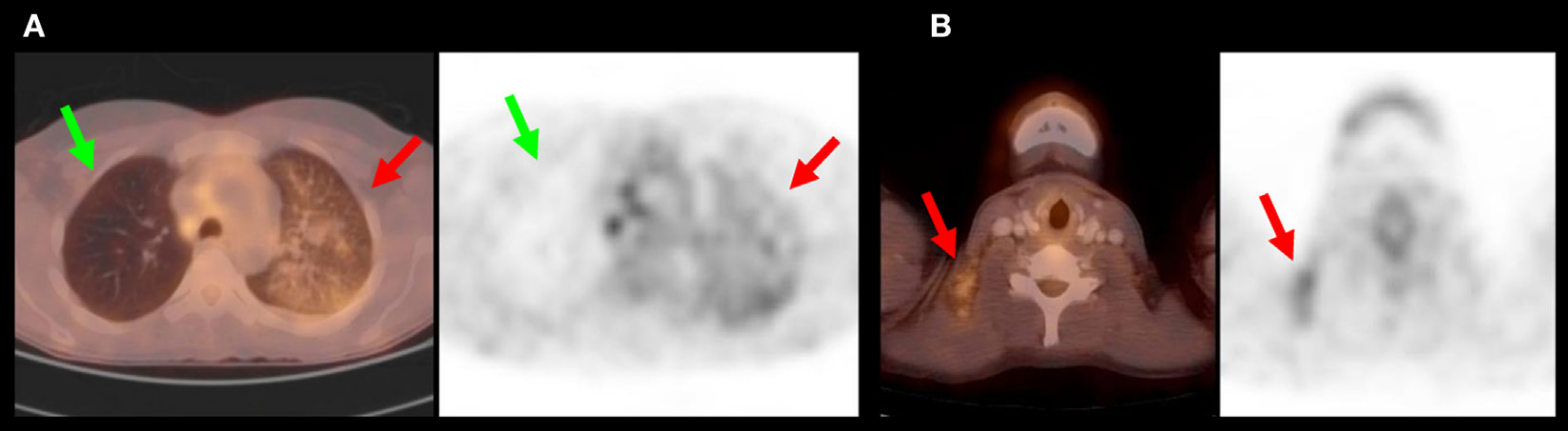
This 36-year-old woman had a thrombosis of her left leg due to a coagulopathy and suffered multiple embolic ischemic strokes. Neither ultrasound of the carotid arteries, cardiac ultrasound, nor cerebral angiography revealed any causative pathology. (A) PET/CT revealed a diffuse, blotchy enhancement of the left lung suggestive of a bronchoalveolar carcinoma (red arrows). Green arrows depict the healthy right lung. The PET/CT overlay is shown on the left side, with the conventional PET-scan on the right. (B) Multiple suspicious lymph nodes were identified in the neck. A biopsy revealed an adenocarcinoma.
